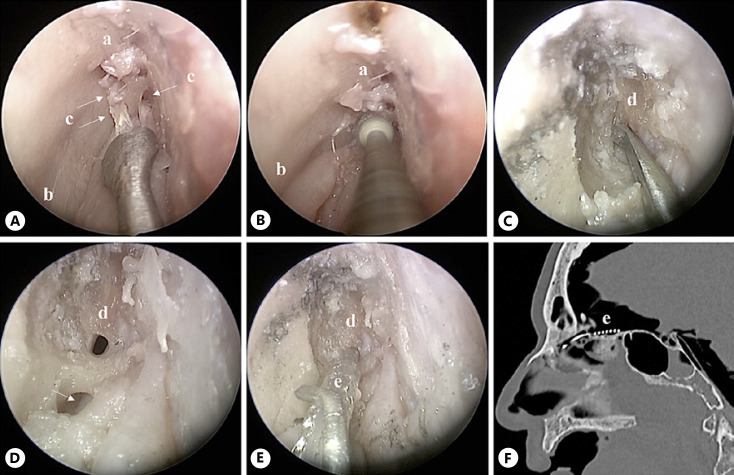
Endoscopic intracranial approach through the cribriform plate (approach 3), right nasal fossa. A The olfactory cleft is prepared; olfactory fila are cut. B, C The cribriform plate is drilled until the dura is reached. D A small hole in the dura is made. E The electrode is inserted inside the hole. F Sagittal CT scan showing the intracranial positioning of the electrode close to the OB. a, olfactory cleft; b, nasal septum; c, arrows, olfactory fila; d, dura; e, electrode.
